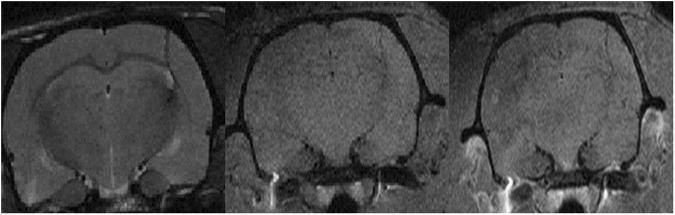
MRI evaluation of long-term survivals. Upper row: T2w (left), T1w before (center) and 8 min after Gd injection (right) images of one of the long-term survivals. No substantial damage was observed with the exception of a scar in the tumor injection site. No tumor presence was observed.
